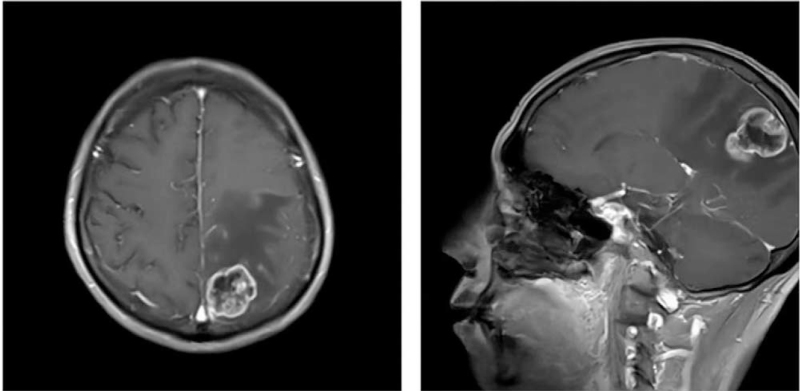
An irregular mass in the left parietal lobe. An uneven T1-weighted low signal and irregular ring enhancement were seen after an enhanced scan of approximately 41 × 26 × 31 mm. The left ventricle was compressed, and the midline structure was shifted to the right.
